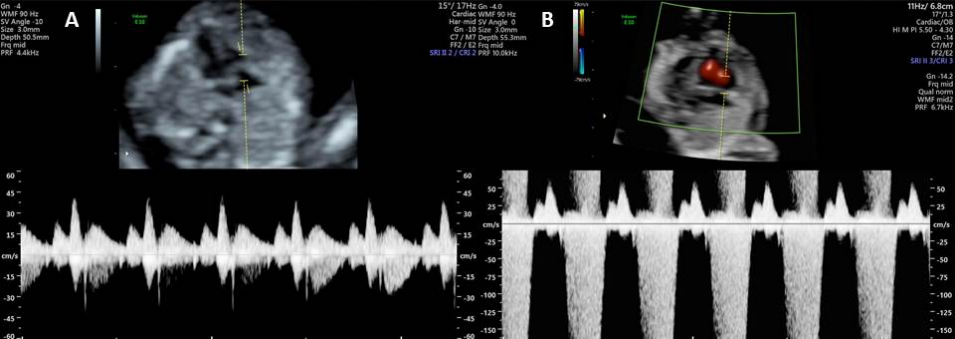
Color and pulsed Doppler examination across the tricuspid valve. Doppler velocity waveforms across the tricuspid valve at 13 weeks of gestation in a normal fetus (A) and in a fetus with trisomy 21 with severe tricuspid regurgitation (B).
